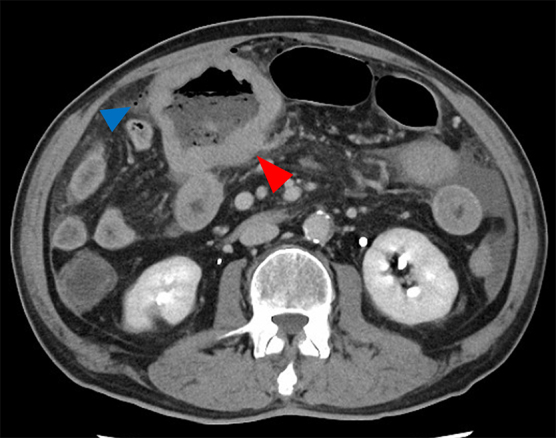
Contrast-enhanced CT at the first medical examination. The red arrow indicates dilatation and thickening of the wall of the intestine. The blue arrow indicates free air and mesenteric fat stranding in the abdomen. CT, computed tomography.
